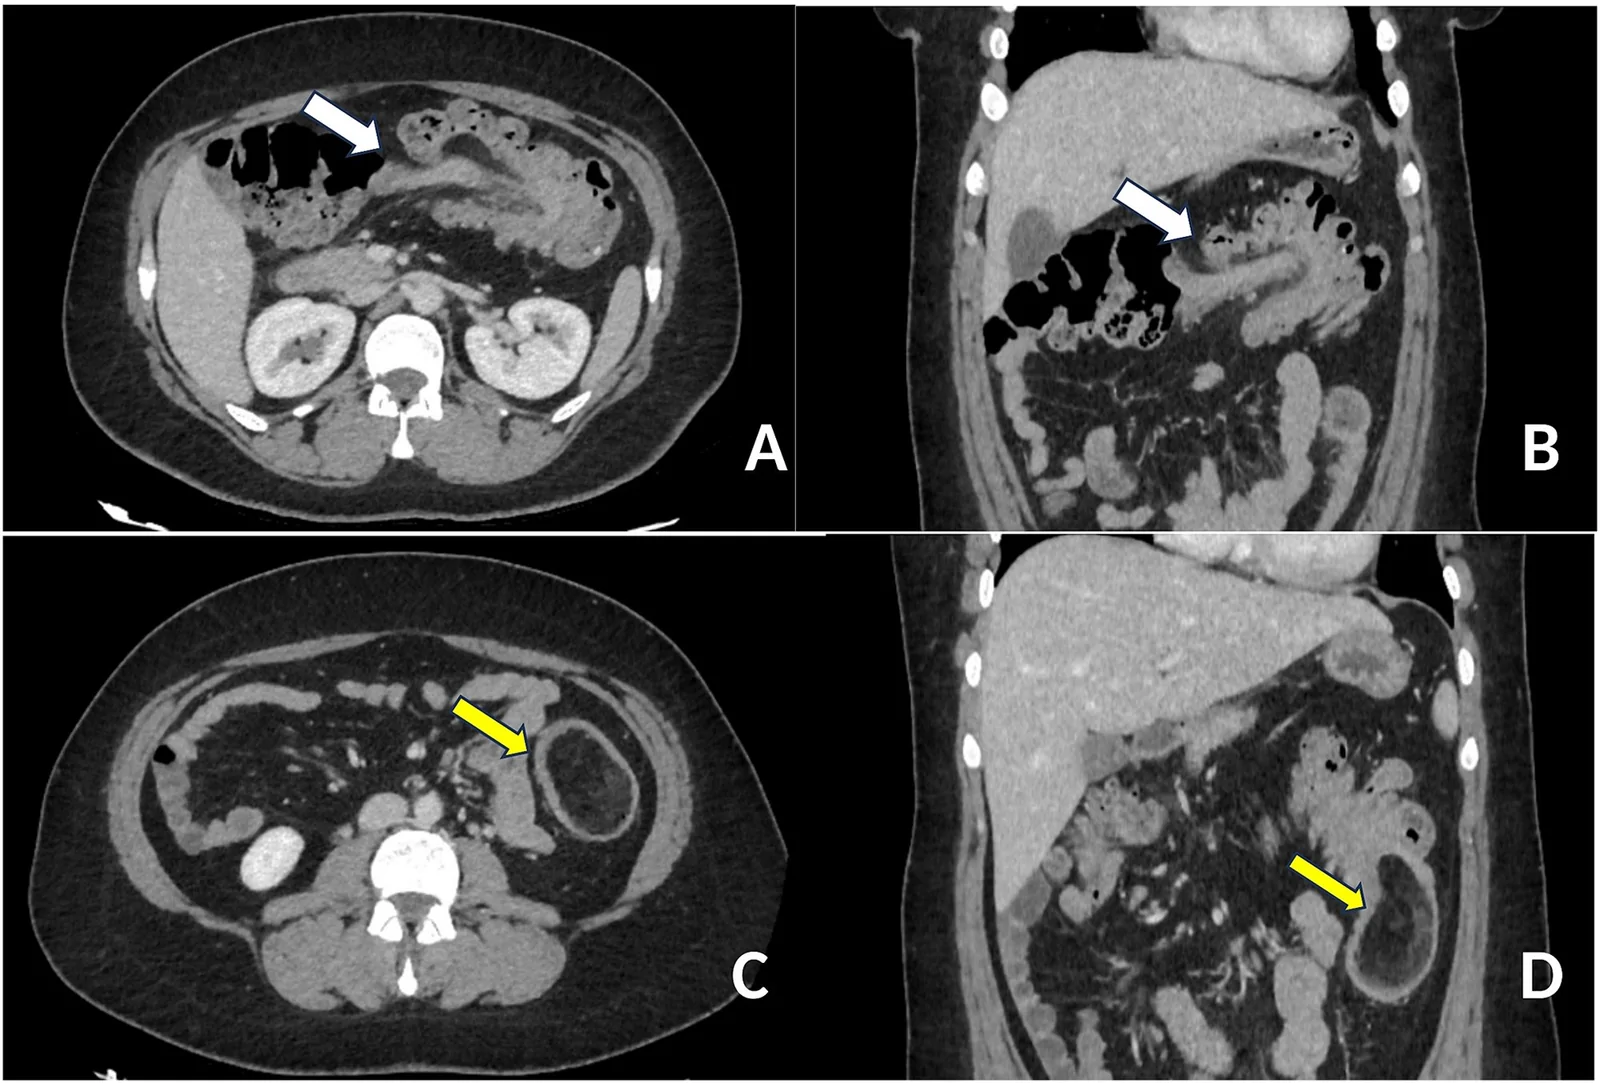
Preoperative contrast-enhanced abdominal CT. Axial (A) and coronal (B) images show the colo-colic intussusception of the transverse colon (white arrow); axial (C) and coronal (D) images show the endoluminal fat-density mass acting as the lead point (yellow arrow).
